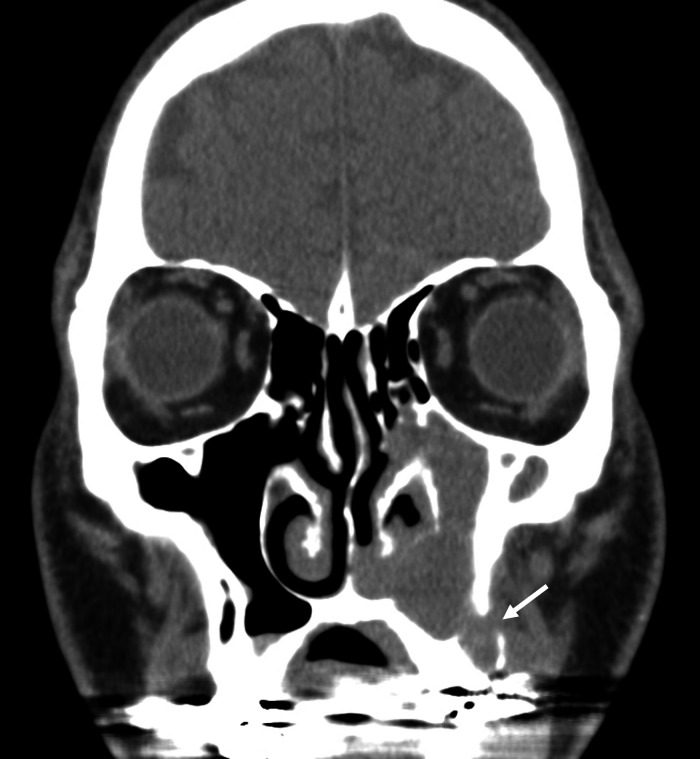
Coronal CT scan of paranasal sinuses in the second case. Coronal CT scan image showing soft tissue lesion in the left maxillary sinus, with thinning and discontinuity of the outer bone wall at the base of the maxillary sinus (arrow).
